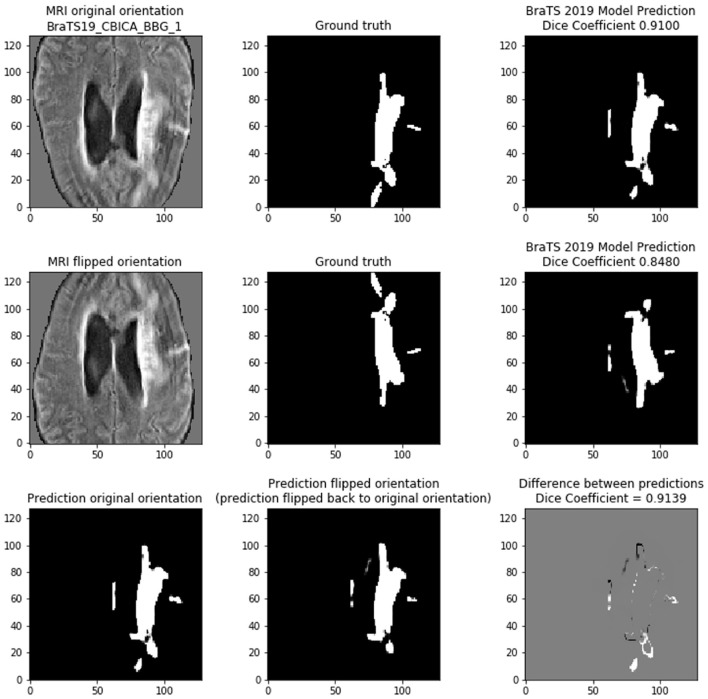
Demonstrating the variability of the 2D BraTS model. (Top) Prediction based on normal orientation of the MRI input. (Middle) Prediction based on vertical flip of the MRI input. (Bottom) Comparing the predictions of the normal and flipped inputs. The prediction of the flipped input was re-flipped to allow direct comparison with the normal orientation prediction. In bottom right figure, gray pixels indicate no difference, black pixels are in the flipped prediction but are not present in the normal prediction, and white pixels are in the normal prediction but are not present in the flipped prediction.
